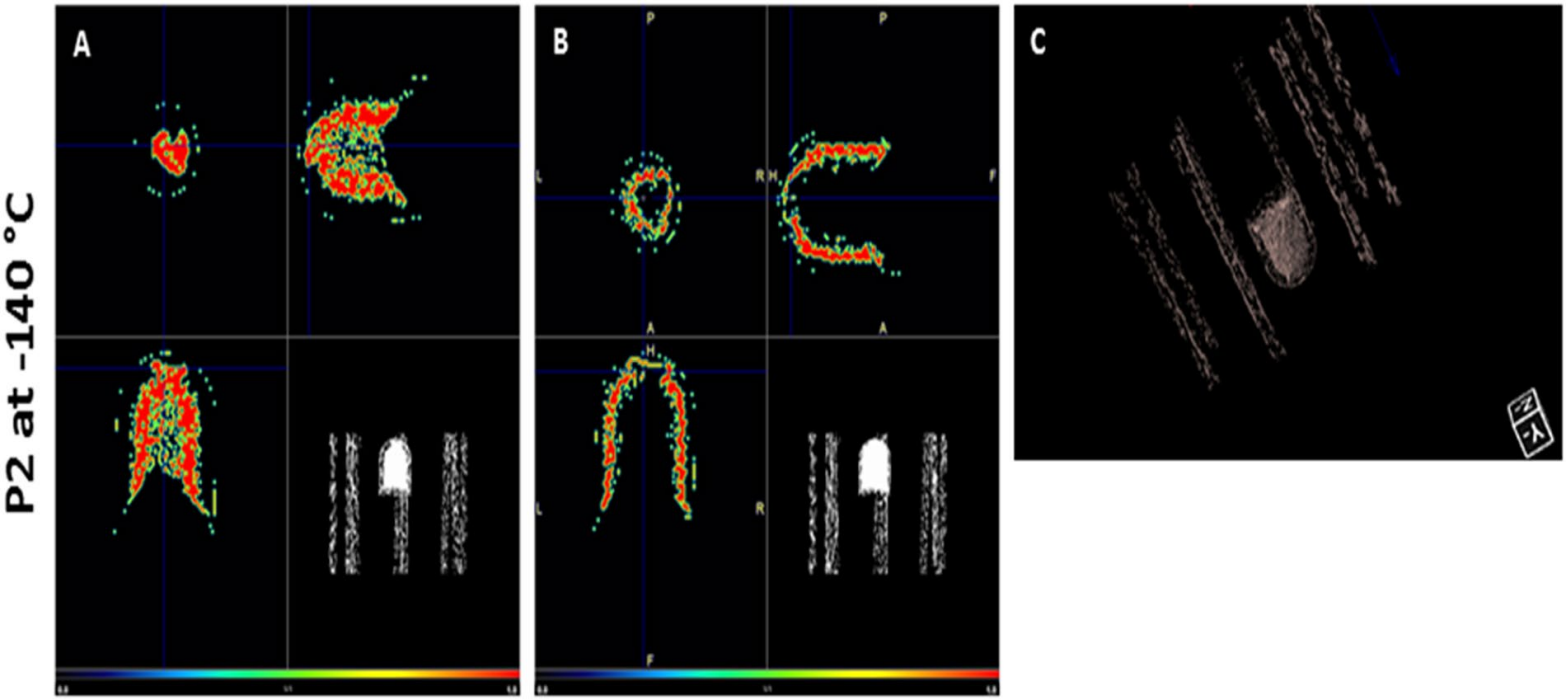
Segmented computed tomography image. Computed tomography image obtained from one sample at −140 °C. (A) Segmentation of the CT image showing only part of the picture with a threshold value from 2.6 to 2.8 CT (on a scale of 0.0 to 1.0), which corresponds to a concentration of 38.2% to 44.4% v/v DMSO. The tissue can be distinguished at the bottom of the cryovial with a similar concentration to the medium. (B) Image obtained under the same conditions as in A, but with a threshold value from 2.8 to 3.0 CT, which corresponds to a concentration of 44.4% to 50.6% v/v DMSO. Note the effect at the borders, suggesting higher concentrations than the solution in the middle. (C) 3D images obtained with the same threshold value as in A. CT: cycle threshold.
